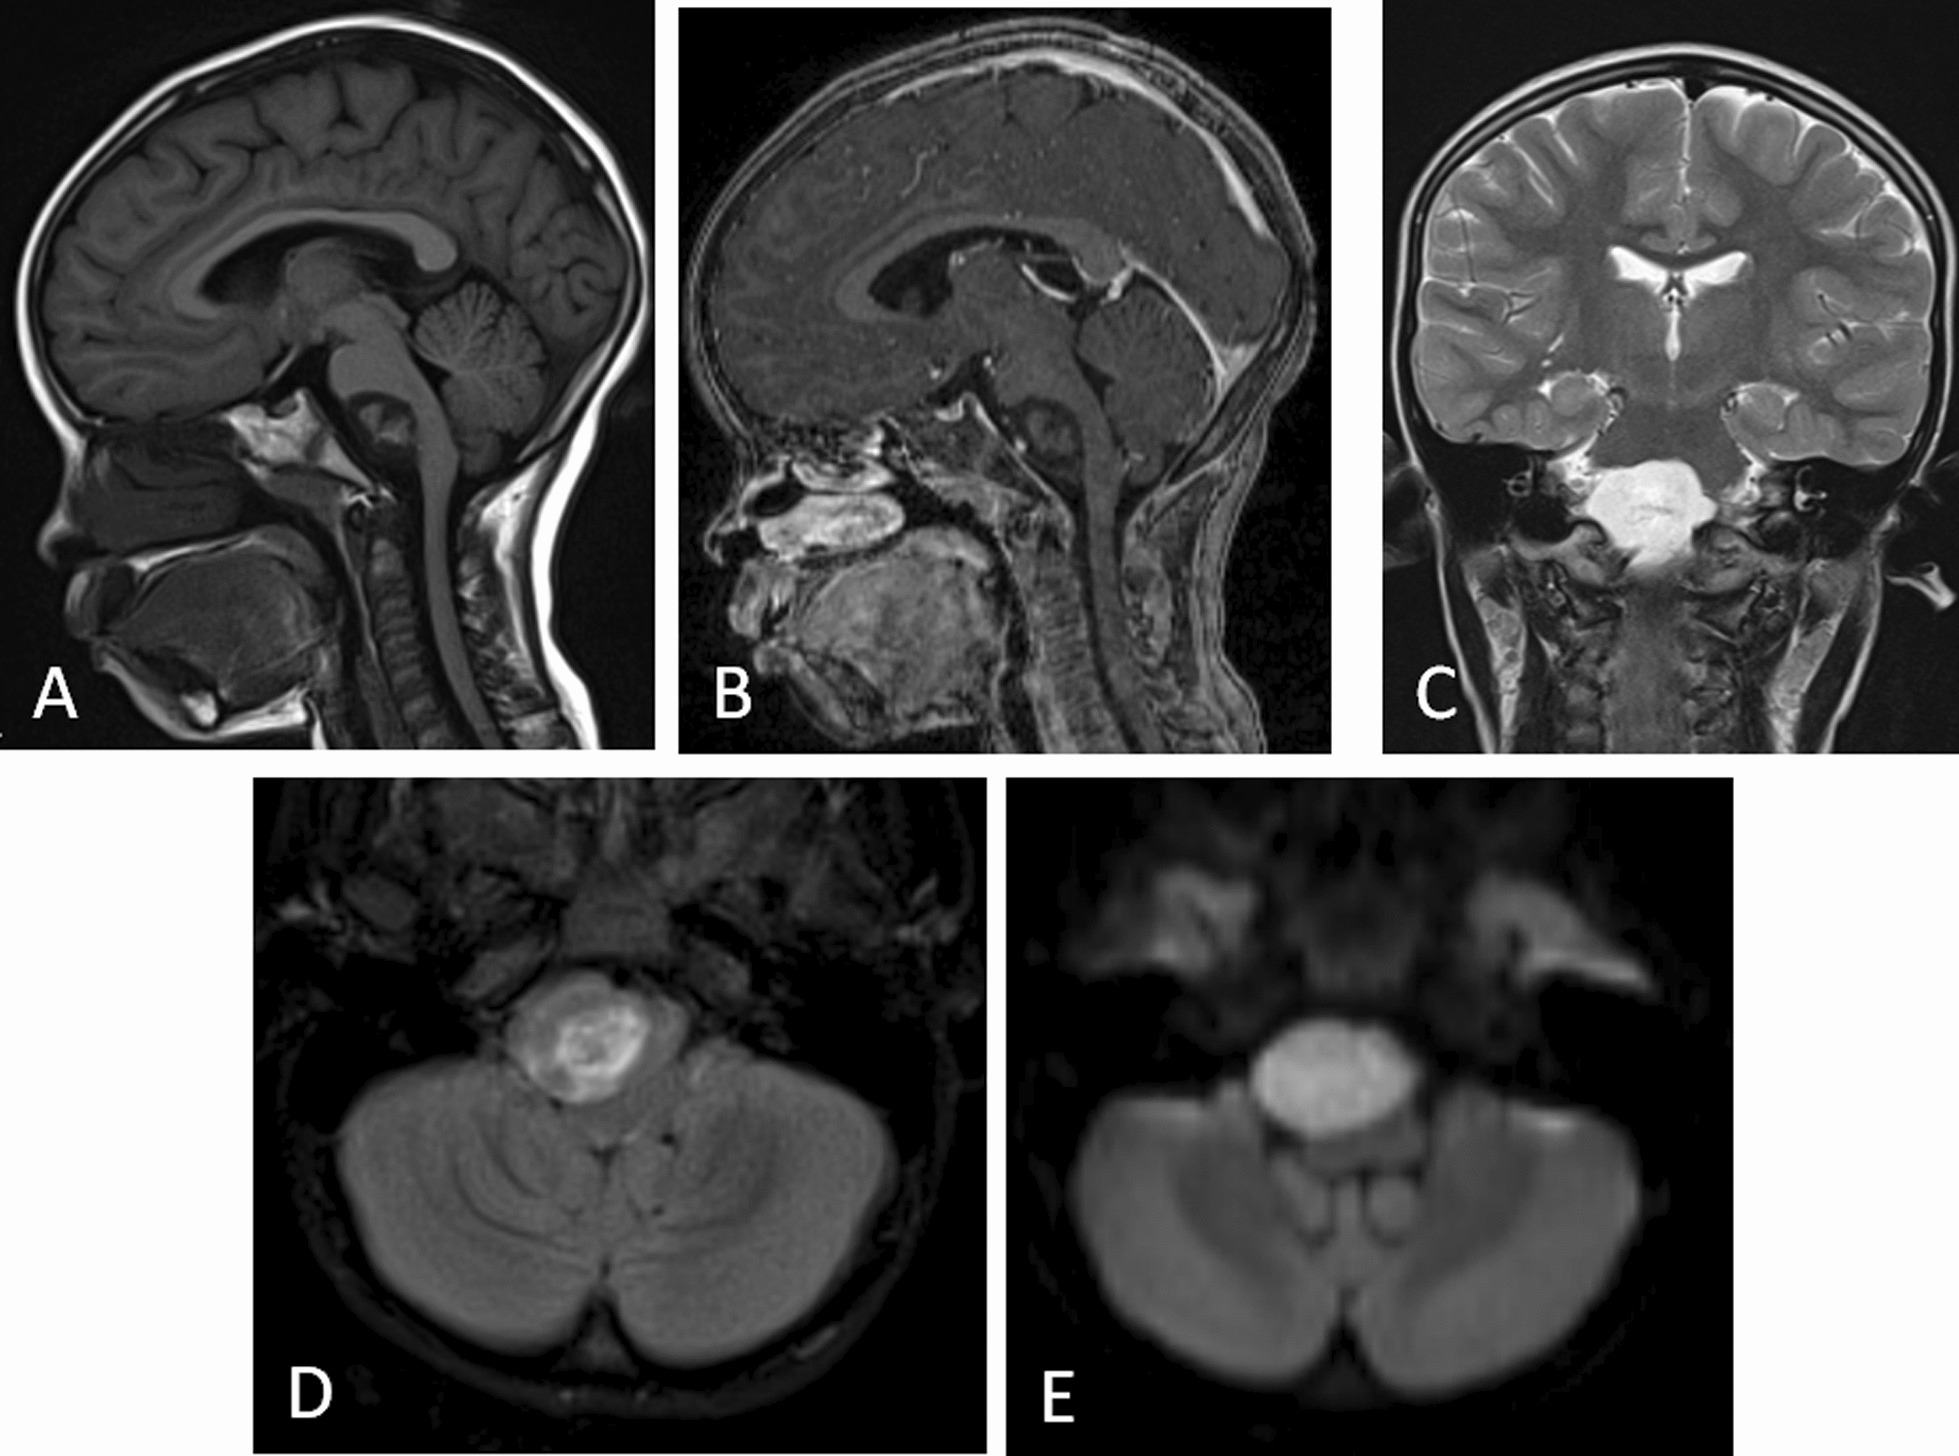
MRI with contrast. A Sagittal T1 reconstruction; prepontine extraaxial mass with hypointense behavior. B Sagittal T1 reconstruction with contrast; no enhancement of the mass observed. C Coronal T2; mass with intensity similar to cerebrospinal fluid (CSF). D Axial FLAIR scan showing lobulated heterogeneous predominantly hyperintense mass giving the appearance of “dirty FLAIR”. E Axial view on DWI showing bright mass
